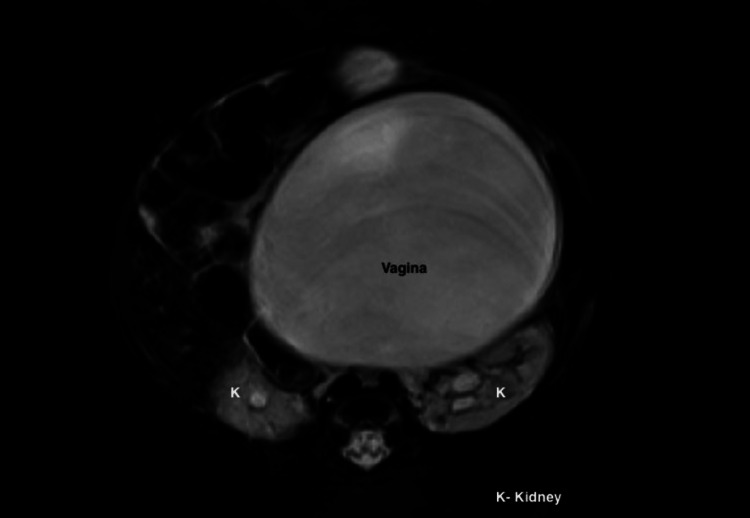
MRI in the axial plane showing a distended vagina containing fluid and bilateral hydronephrosis.
